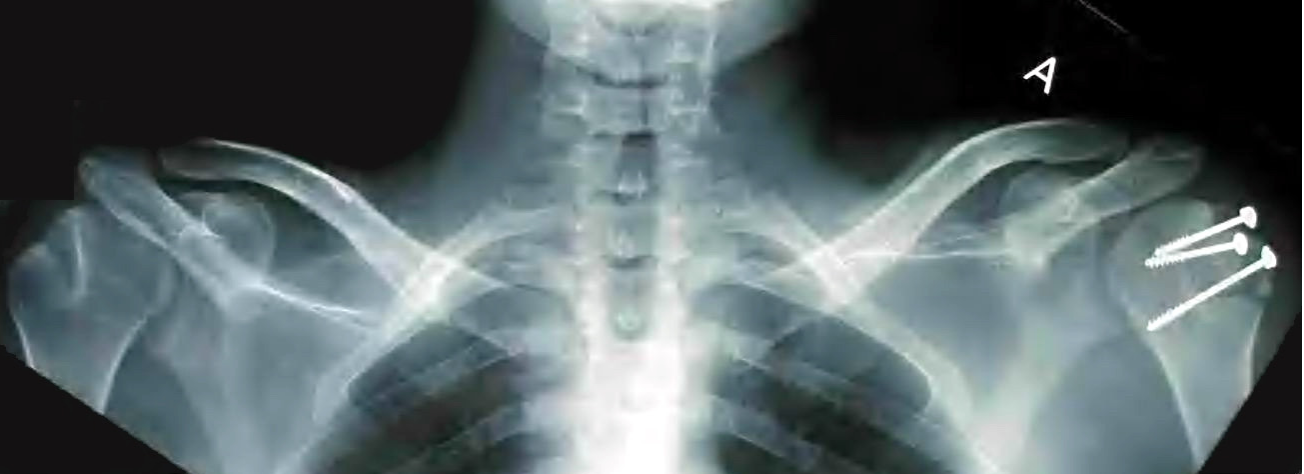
Post surgery radiographic control.
